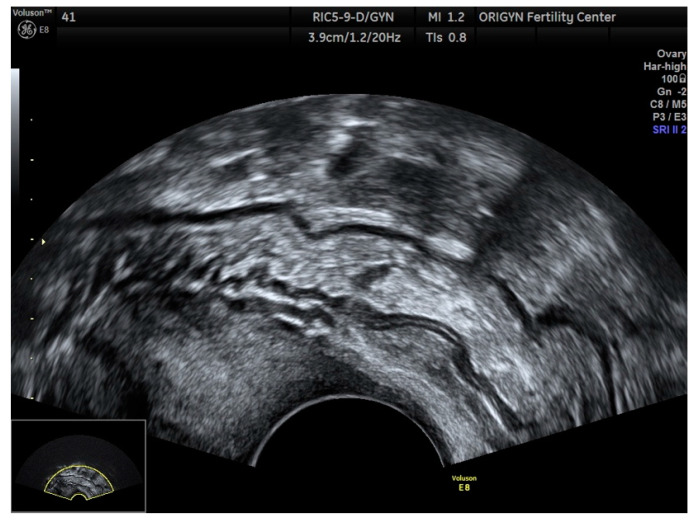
Ultrasound appearance of a normal bowel loop.
